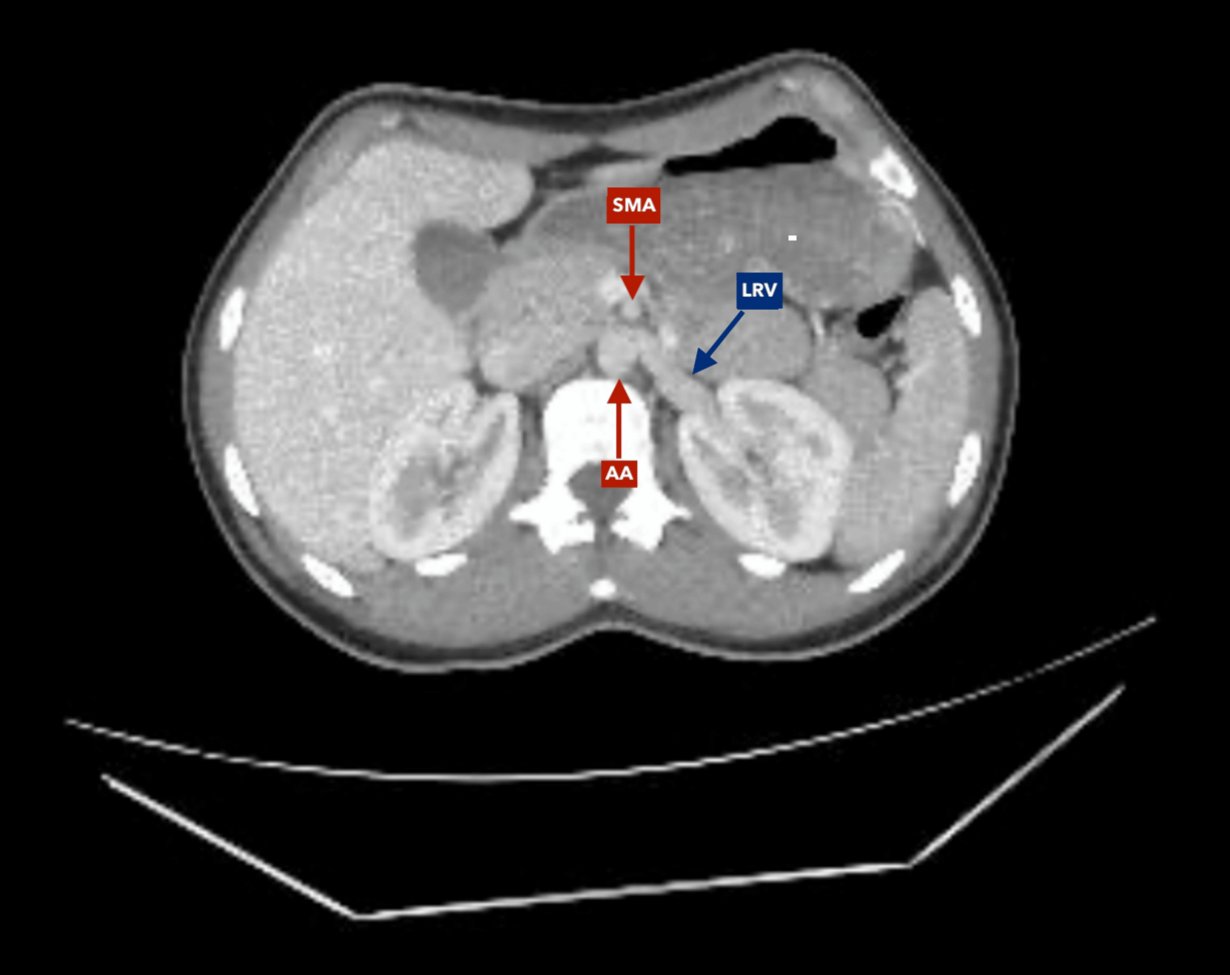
Contrast-enhanced computed tomography of the abdomen, axial reconstruction, venous phase Nutcracker syndrome. Stenosis secondary to compression of the left renal vein (LRV) by the superior mesenteric artery (SMA) over the abdominal aorta (AA).
